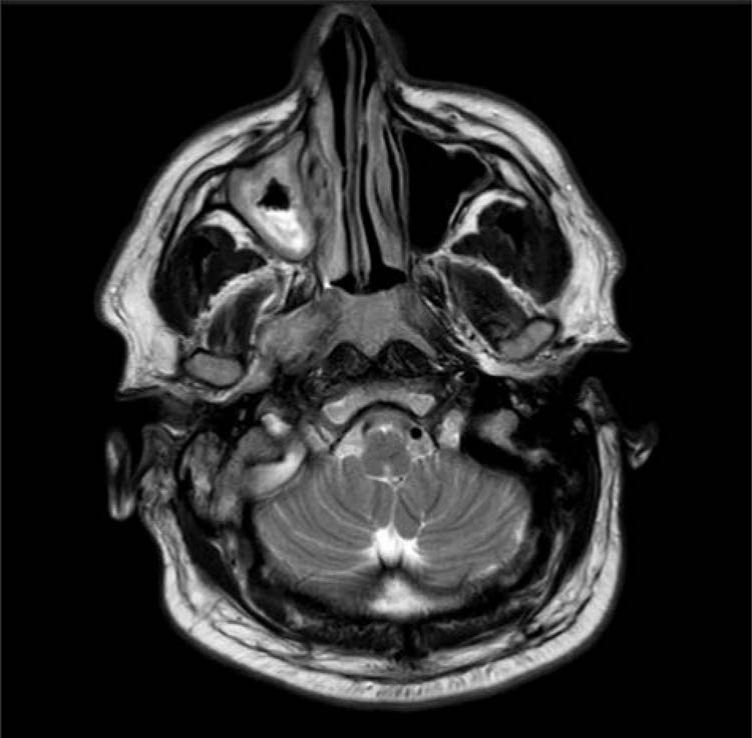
Axial MRI image showing high attenuation lesion affecting the right skull base.
